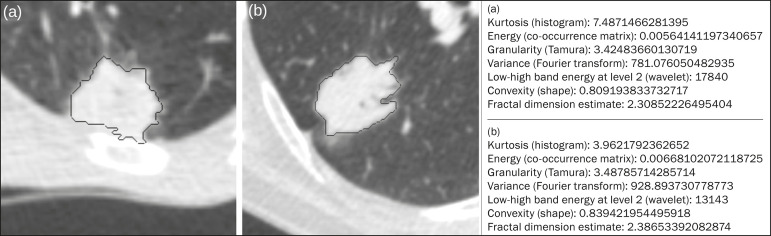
Examples of tumors on axial CT images with lung window settings and their respective radiomic feature values. a: Lung adenocarcinoma in a 68-yearold male patient who evolved to death (overall survival, 55 days). b: Lung adenocarcinoma in a 77-year-old female patient who was still alive at this writing (overall survival, 2801 days).
